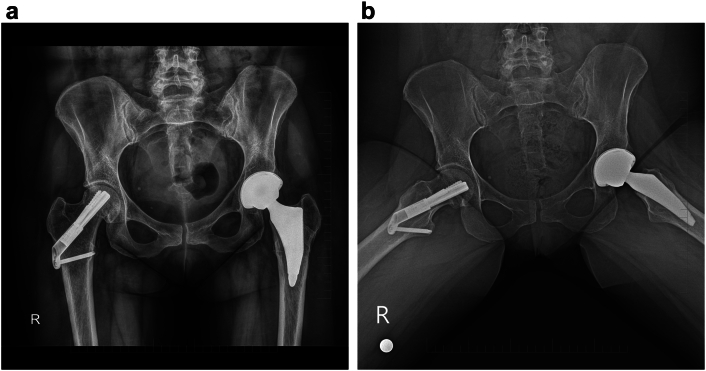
Radiographs 4 years after total hip replacement performed for avascular necrosis of the femoral head. 5a, - Anteroposterior (AP) view of the pelvis. 5b, - Axial view of the pelvis.
